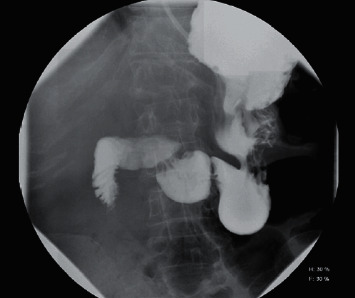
X-ray small bowel follow-through partial obstruction in the 2^nd^ portion of the duodenum as evidenced by narrowing without contrast follow-through.
